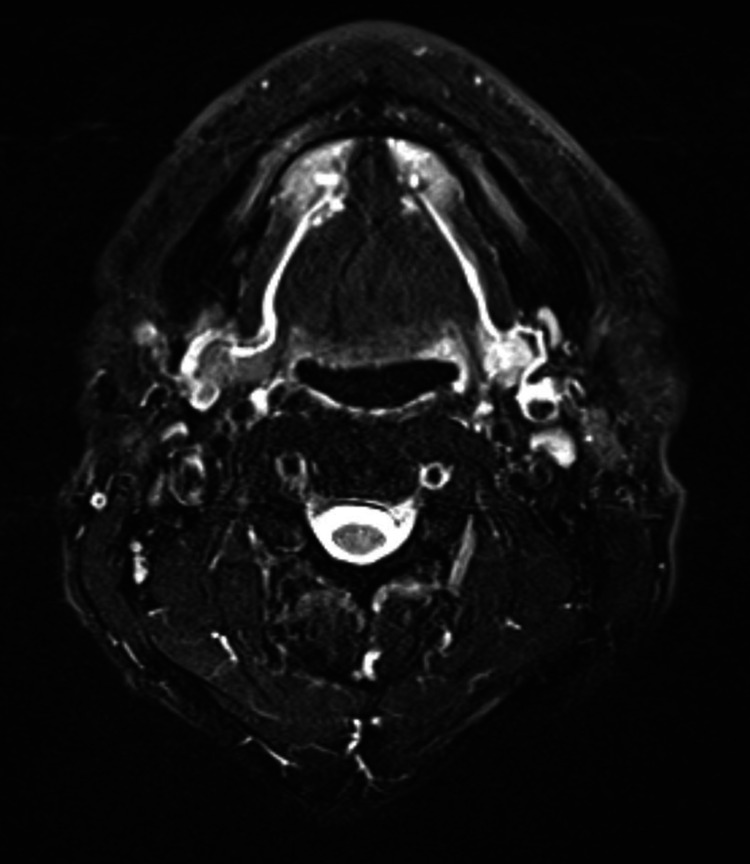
T2WI(FS) MRI one year and four months after the implant. Detection of Wharton’s duct and the submandibular gland. T2WI(FS): T2-weighted fat-suppressed; MRI: magnetic resonance imaging
